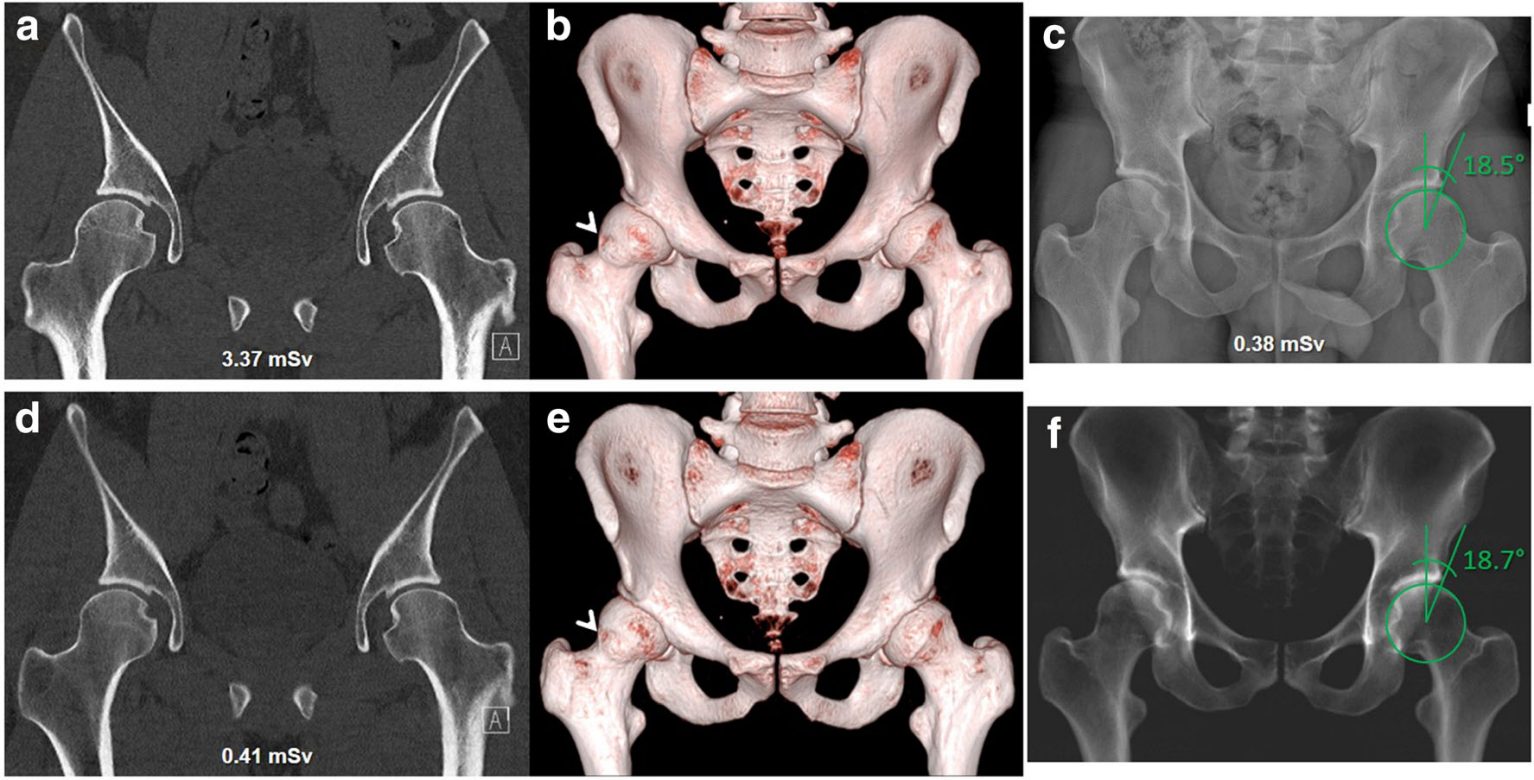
A 25-year-old male with symptomatic hip dysplasia of both sides. Reformatted coronal CT image of both hip joints, scanned with the standard protocol (a) and ultra-low-dose protocol with tin filtration (Sn140kV/50mAs; d) show clear depiction of anatomy. 3D VRT pelvic reconstruction of the standard CT (b) and ultra-low-dose CT (e) both demonstrate clear pelvic anatomy. Calculated virtual pelvic a.p. radiograph of the ultra-low-dose CT (f) shows clear depiction of anatomy compared to the digital a.p. radiograph of the pelvis (c). Measurement of the left center-edge angle was almost identical with 18.7° for the virtual and 18.5° for the digital radiograph. Note cam deformity of the right femur in b and e (arrowheads)
